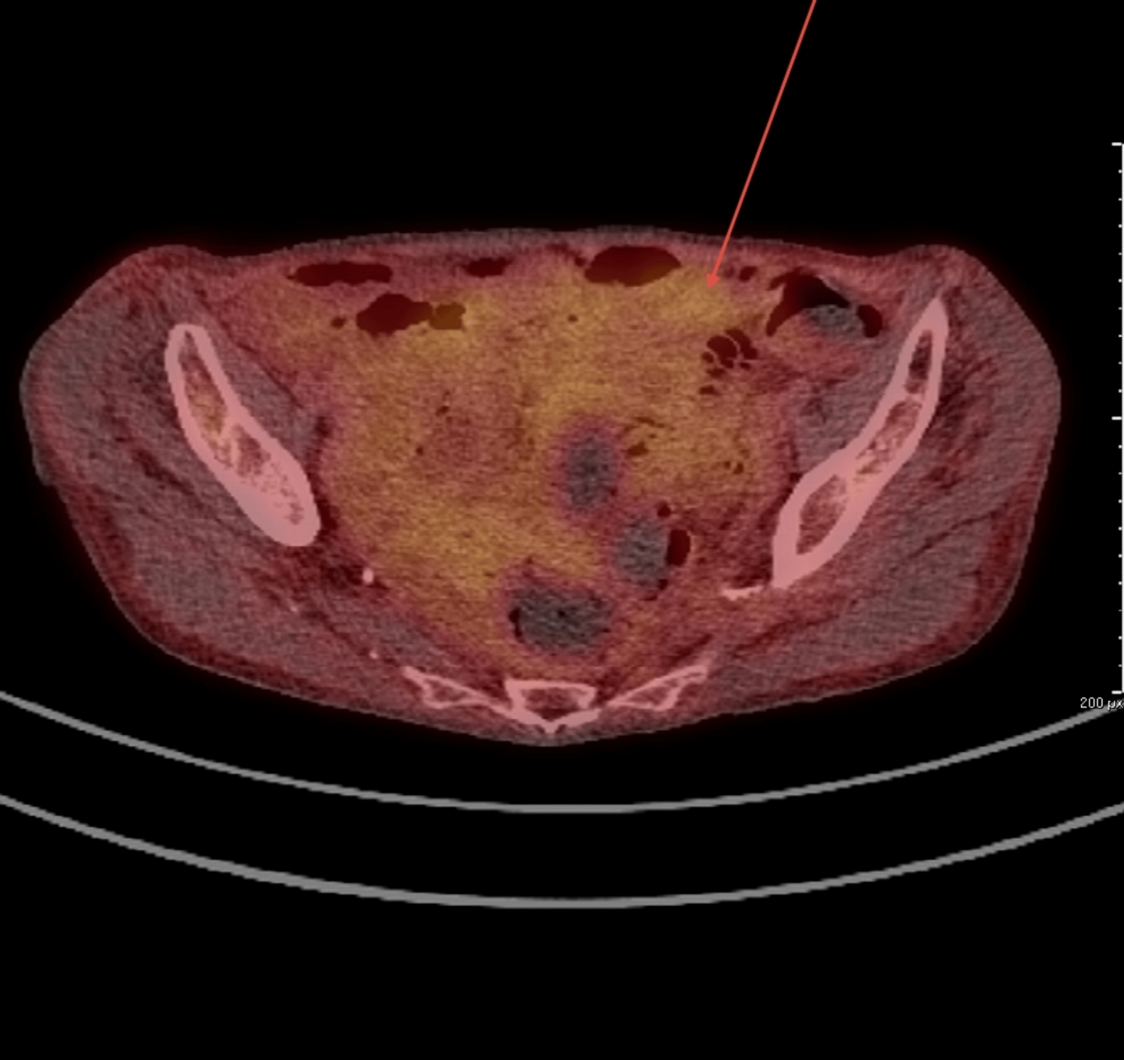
CT PET showing low-grade uptake in the bowel, with multiple, small, poorly avid mesenteric lymph nodes This indicates a high likelihood of developing malignancy. PET: positron emission tomography
